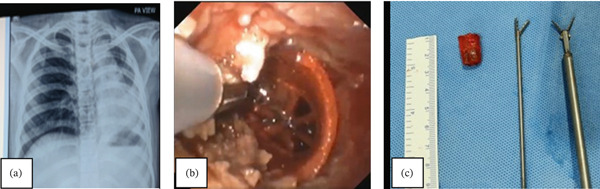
(a) Chest radiograph showing atelectasis of left lung. (b) Granulation removal using cryoablation. (c) Retrieved pen cap with rigid forceps.
